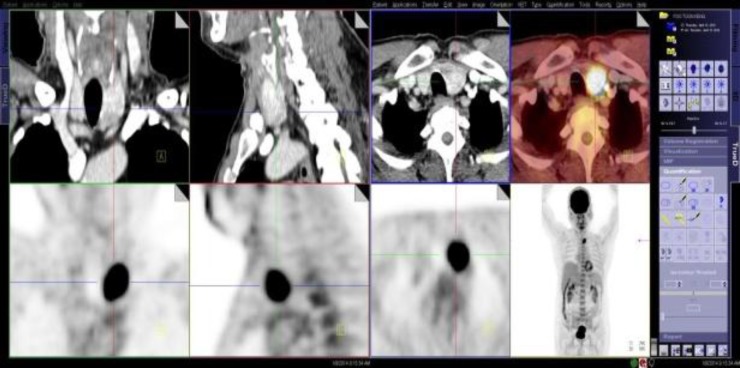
Multiplanar (coronal, sagittal and axial) and MIP images on CT and PET/CT (upper row) and on PET (lower row) demonstrating a subtle enlarged left thyroid lobe from a heterogenous enhancing lesion seen on CT. The lesion corresponds with high 18F-FDG intensity uptake
